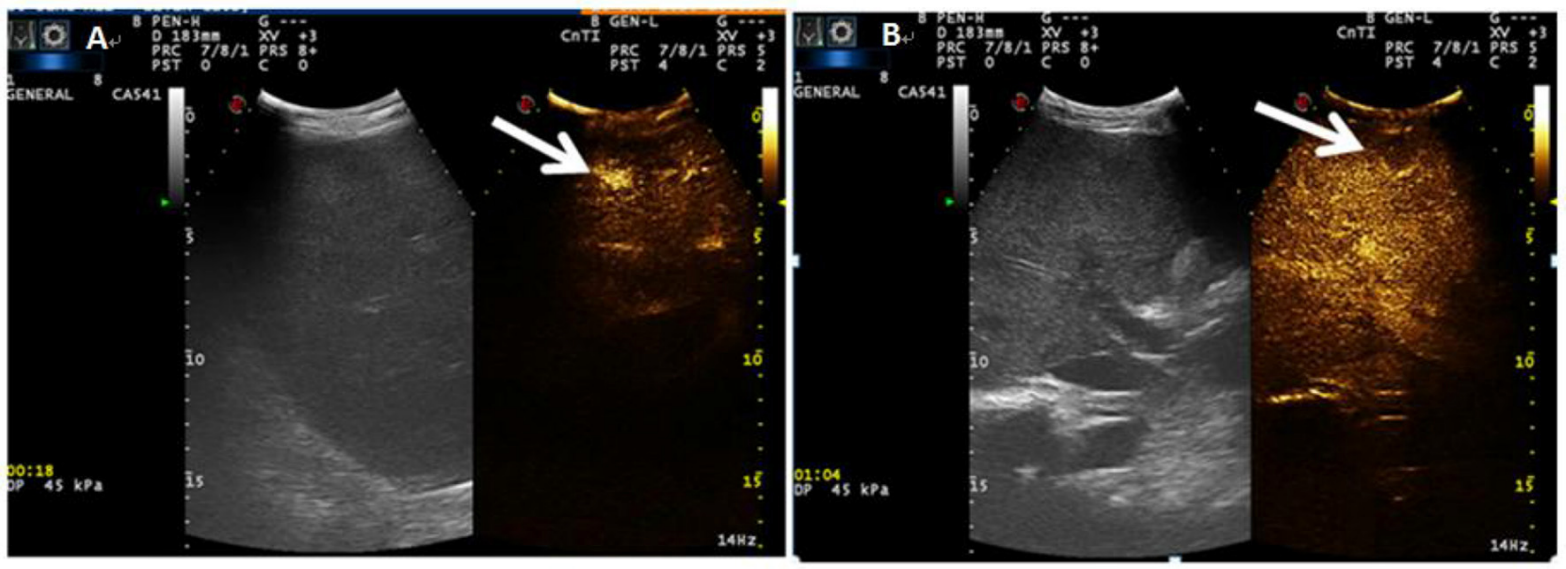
(A) Indicates irregular hyper-enhancement in the arterial phase of the lesion, with a transient abnormal enhancement area (white arrow) in the center. (B) shows the abnormal enhancement area in the delayed phase with low enhancement (white arrow) in contrast-enhanced ultrasound.
